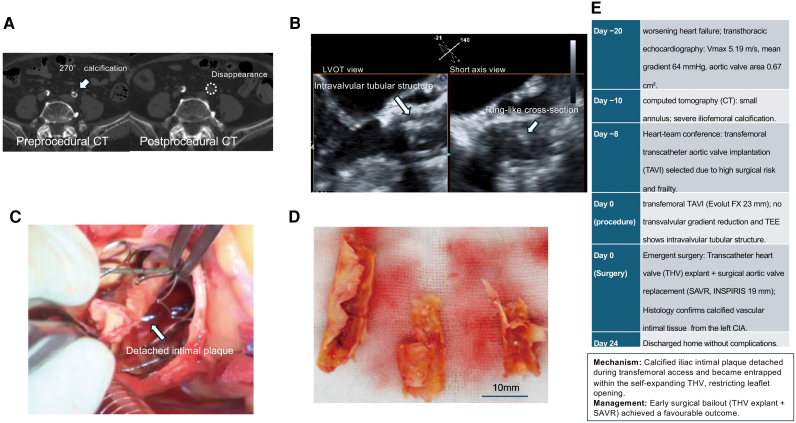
(*A*) Axial CT before and after the procedure showing a 270° circumferential calcified plaque in the patient’s left common iliac artery on preprocedural CT (arrow) and its disappearance on postprocedural CT (dotted circle), consistent with endarterectomy-like detachment. (*B*) Biplane long-axis transoesophageal echocardiography immediately after valve deployment demonstrating restricted leaflet opening due to an intravalvular tubular structure within the self-expanding transcatheter heart valve (arrow indicates the intravalvular tubular structure). (*C*) Intraoperative photograph showing a detached intimal plaque entrapped within the valve frame and mechanically impinging on a leaflet (arrow indicates the plaque). (*D*) Retrieved tubular calcified tissue consistent with vascular intimal origin (left common iliac artery). (*E*) Timeline of key clinical events.
